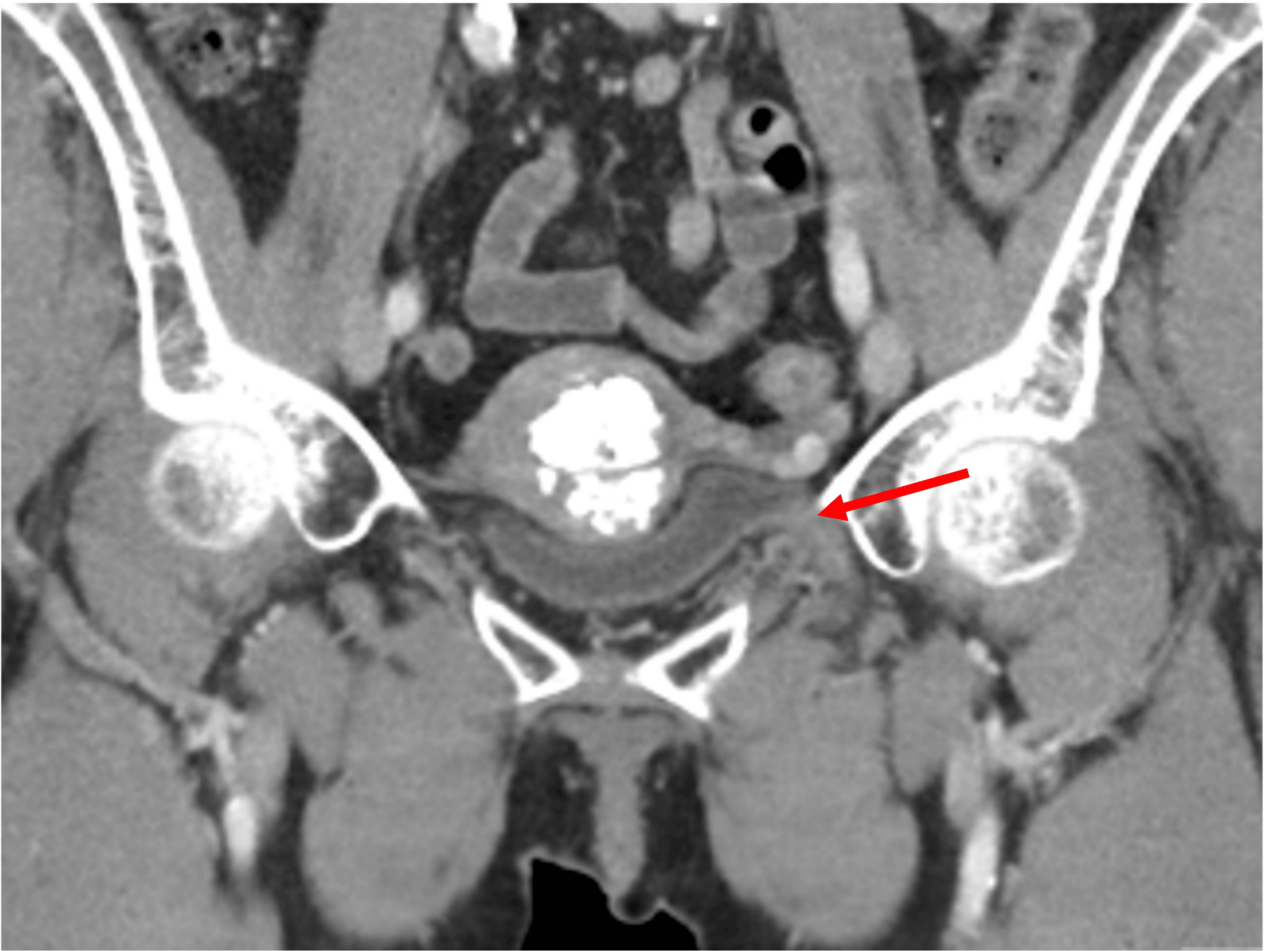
Contrast-enhanced coronal computed tomography image depicting the narrow-neck origin of a bladder diverticulum as it arises from the bladder wall and courses through the obturator foramen.
